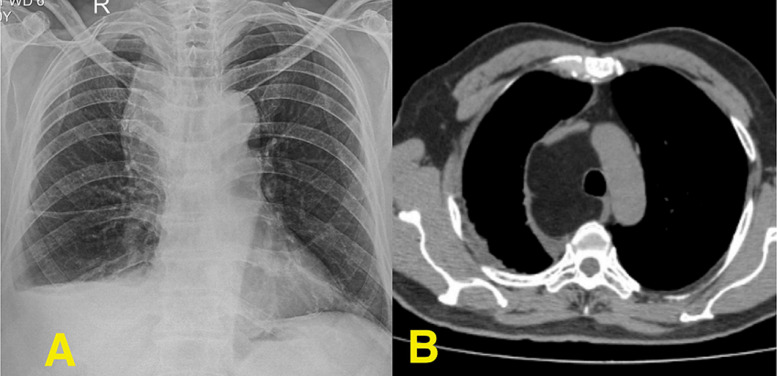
A) chest X-ray showing right pleural effusion with opacity in mediastinum, B) axial section of CECT thorax showing well-encapsulated, homogenous fat-density lesion in the mediastinum
